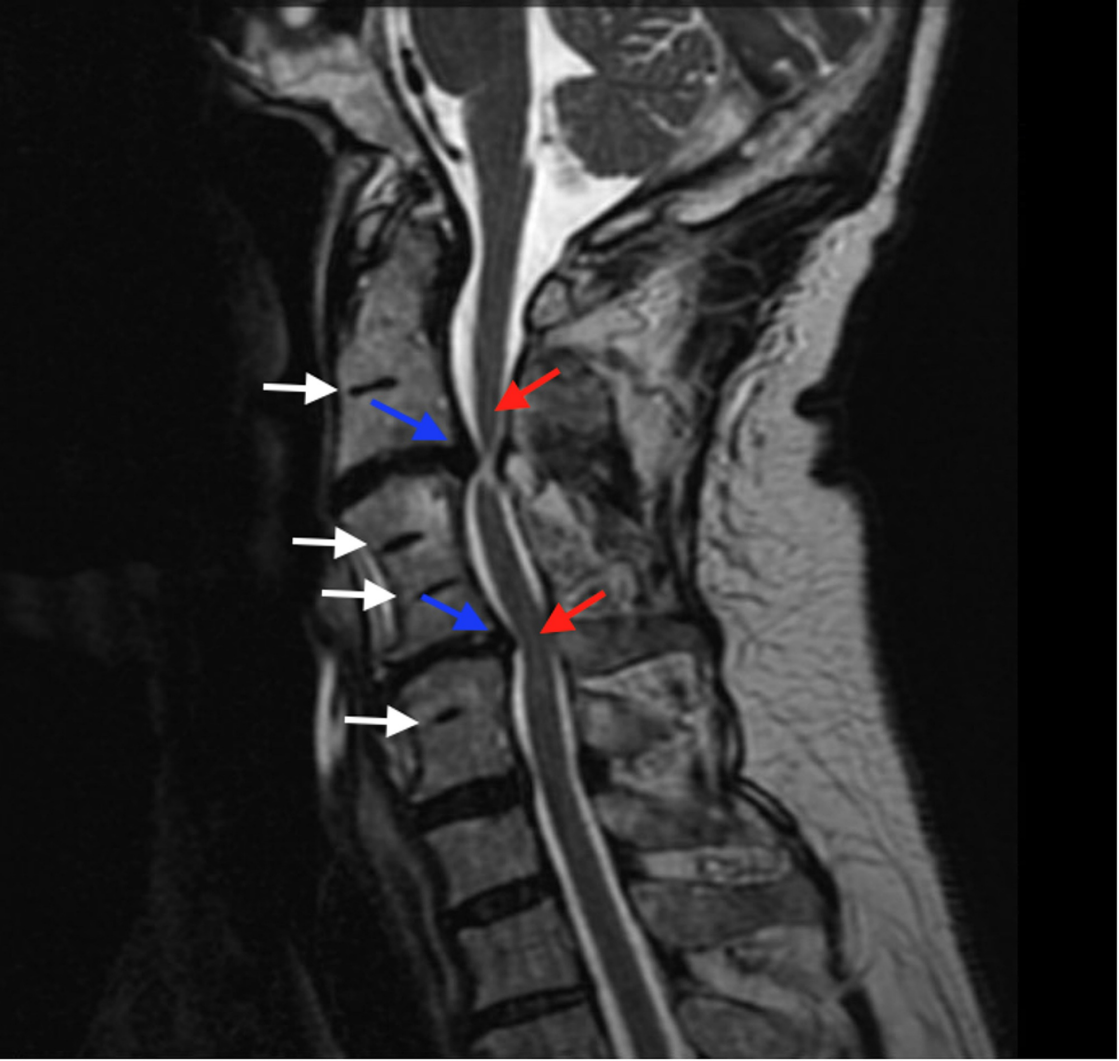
MRI of Cervical Spine. A mid-sagittal MRI T2 sequence of the cervical spine demonstrating multiple vertebral bodies that have auto-fused (white arrows.) Lying between these fused segments are multiple levels of disc bulges (blue arrows) causing significant cord signal change indicative of edema or myelomalacia (red arrows).
